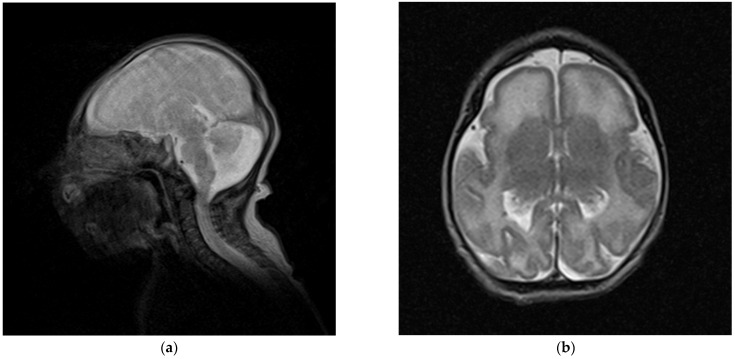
Brain MRI (turbo spin echo T2) performed at 3 days of life. (a) Sagittal view: microencephaly with flat frontal bone and enlarged posterior fossa with large cisterna magna. (b) Axial view: posteriorly enlarged lateral ventricles and fronto-polar lissencephaly with increased cortical thickness.
